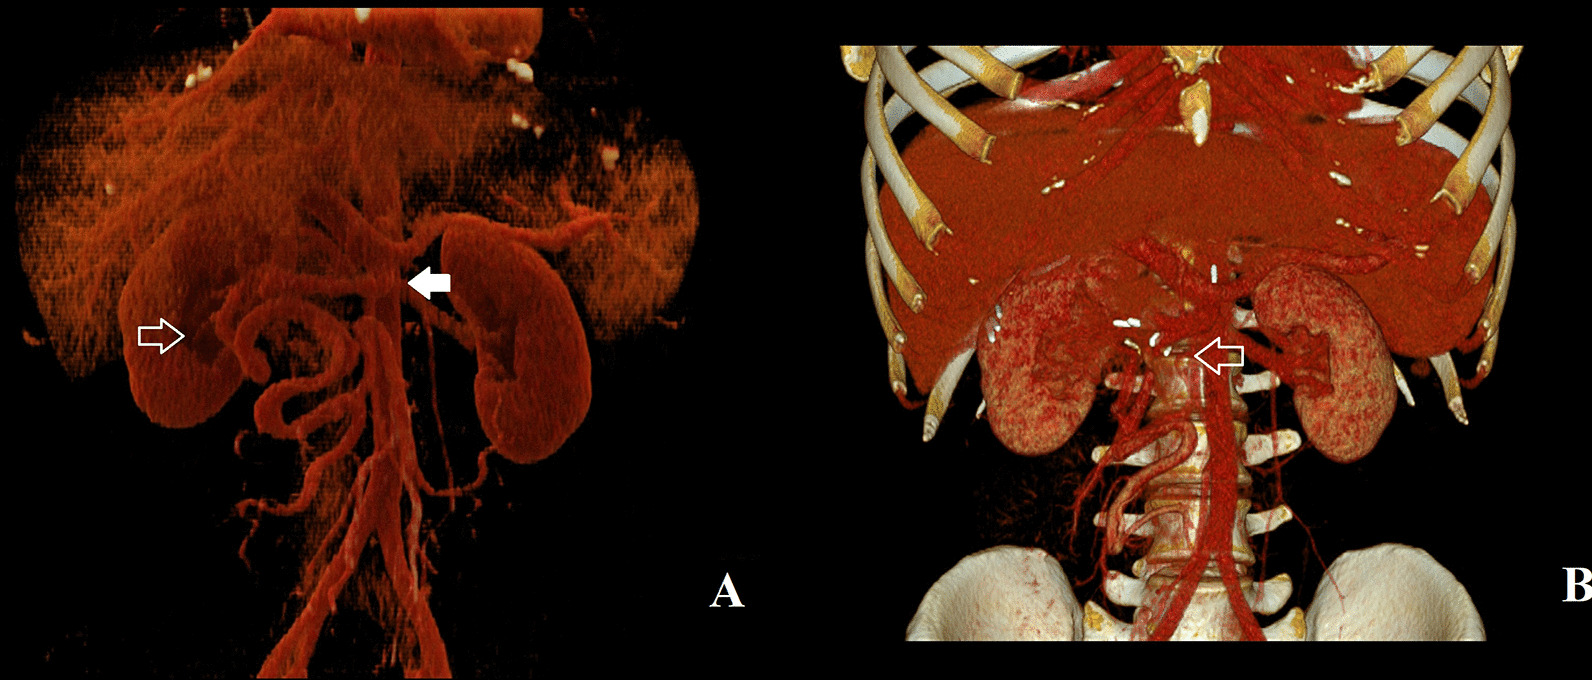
A 3D reconstructions of vascular system from coronal CT scans: A Preoperative CT reconstruction: the full white arrow points to the proximal section of the SMV, the contoured white arrow points towards collaterals running through the duodenum. B Postoperative CT reconstruction shows patent anastomosis between collateral branch from the distal section of the SMV and the anterior inferior pancreaticoduodenal vein
